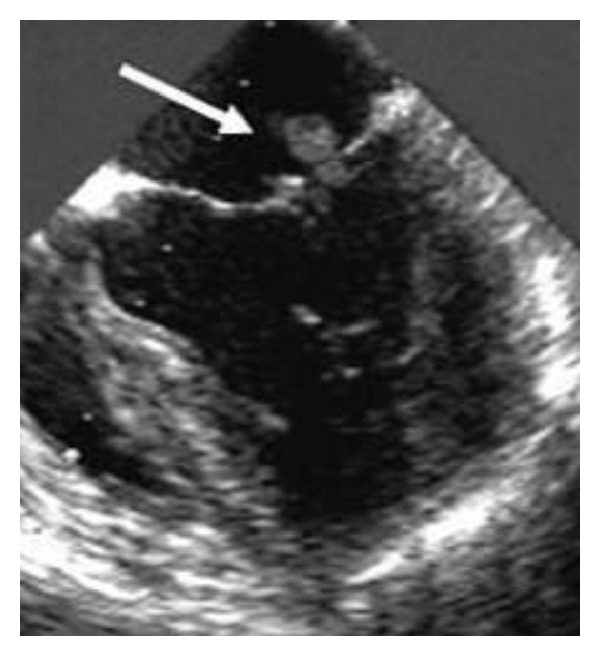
Trans esophageal echocardiogram showing Libman-sacks vegetation.
